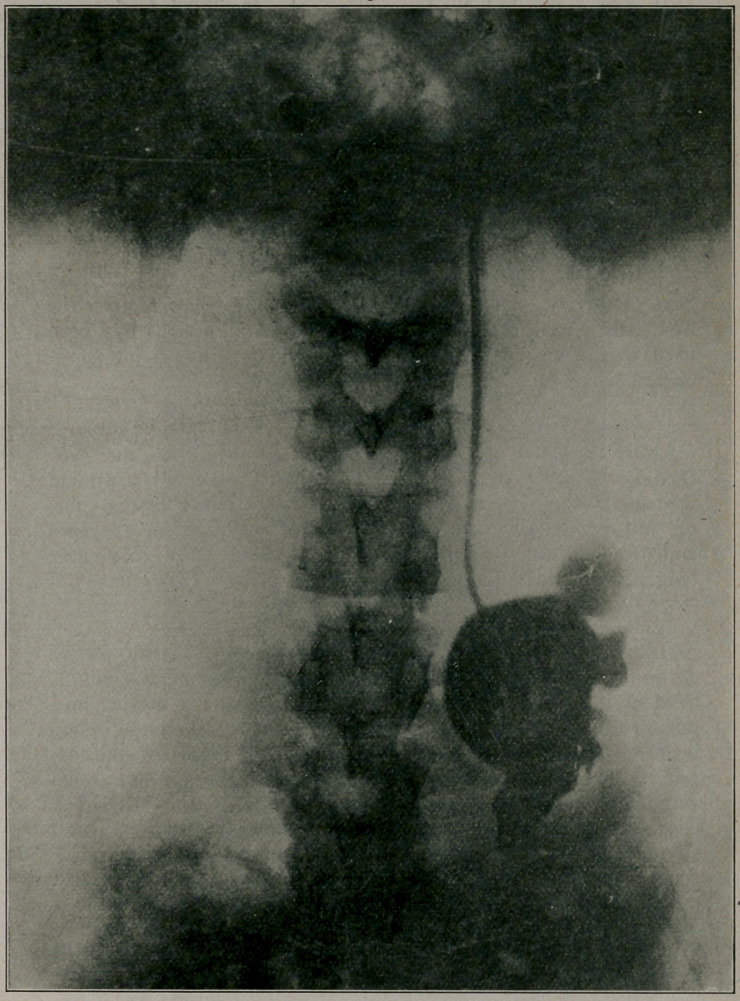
# Pyelography (Radiography of Ureter and Renal Pelvis) in Diagnosis of Surgical Conditions of Kidney
